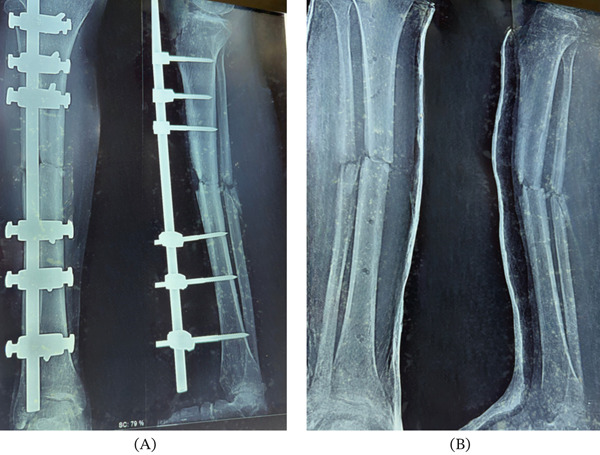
Delayed progression under temporary fixation and preconversion stage. (A) Anteroposterior and lateral radiographs dated January 17, 2025, showing persistent fracture lines and minimal progression toward union while the external fixator remained in situ. (B) Anteroposterior and lateral radiographs dated February 12, 2025, after removal of the external fixator, showing interim cast immobilization before definitive intramedullary nailing.
